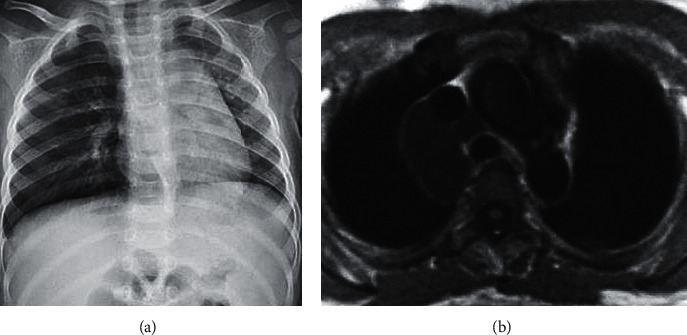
X-ray and MRI image evaluation of a male case (2 years old): (a) a chest X-ray; (b) an MRI image.
